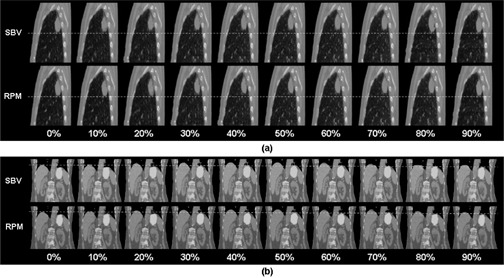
Representative images of 4D ${\text{CT}}_{\text{SBV}}$ and 4D ${\text{CT}}_{\text{RPM}}$ for a lung cancer patient (a) and a liver cancer patient (b). Images are elongated and dash lines are added for better visualization of the respiratory motion.
